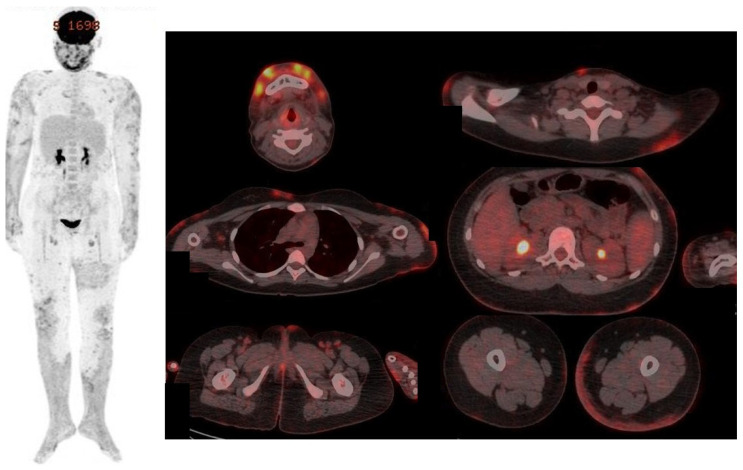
PET-CT image showing subcutaneous soft-tissue strandings and multifocal infiltrations and diffuse and heterogenous FDG uptake throughout the upper and lower extremities, face, trunk, and vulva.
